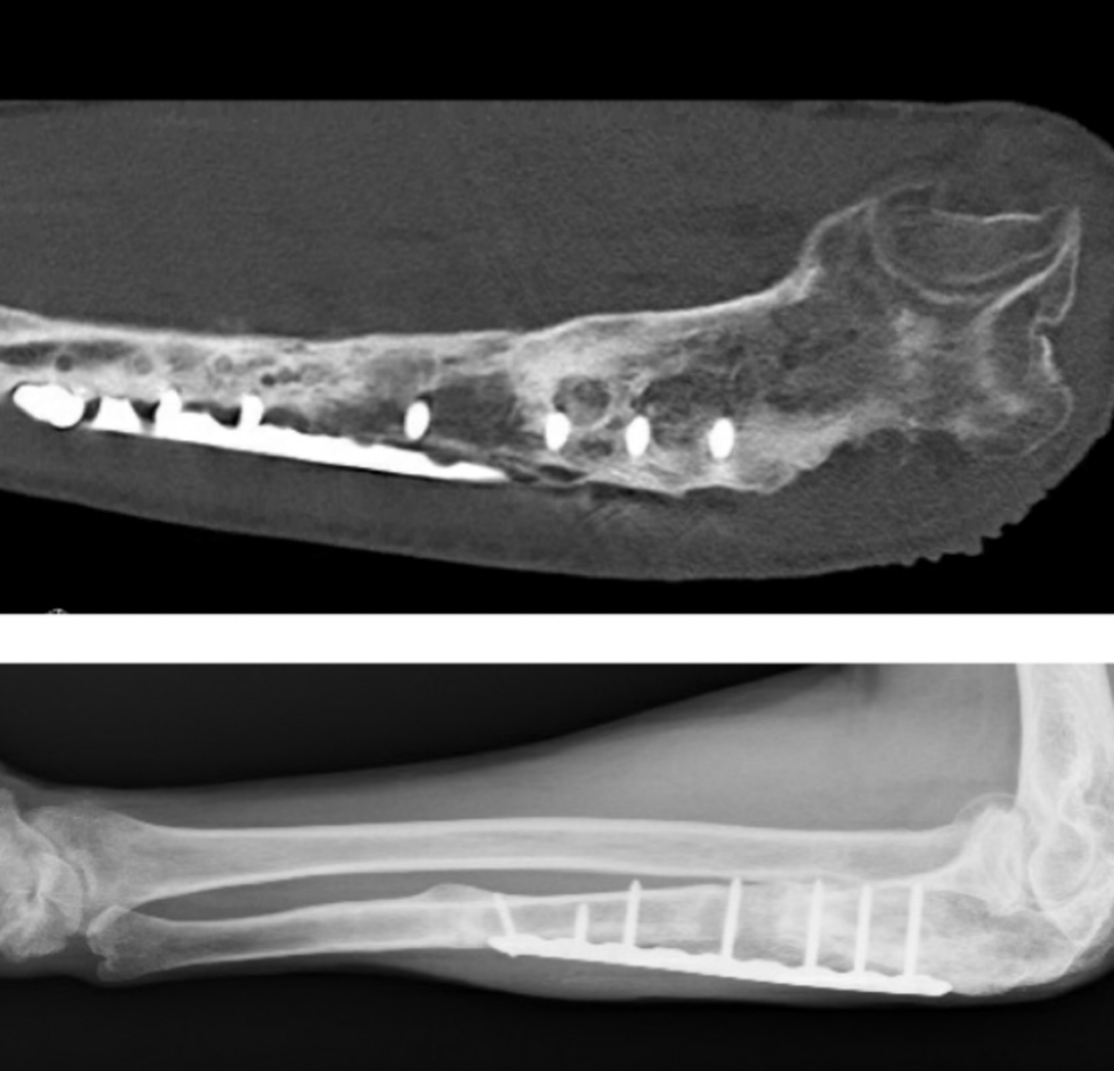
One year later and the patient presented with a fistula X-ray and CT showed complete healing and no signs of osteomyelitis.
